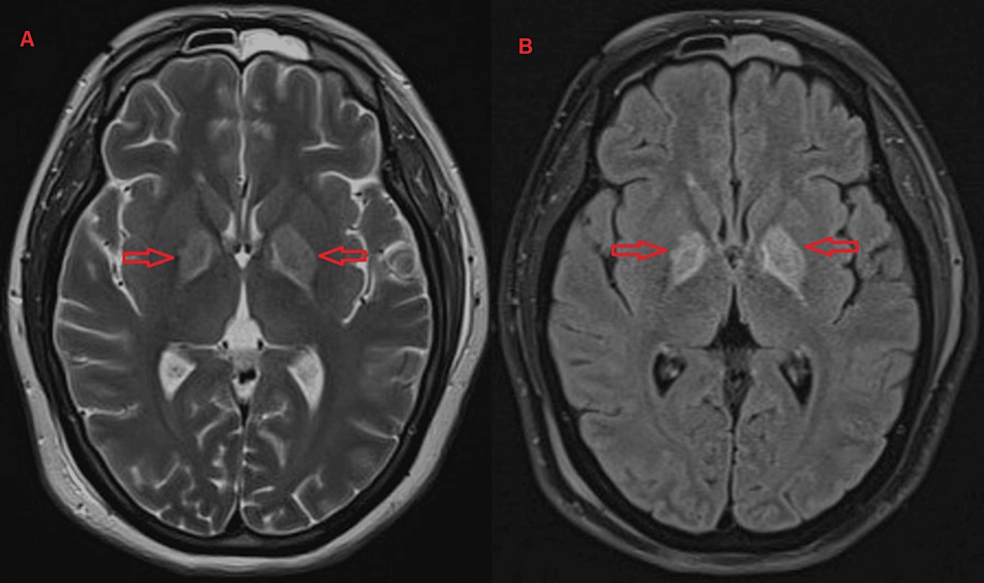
Magnetic resonance imaging (MRI) of the brain of Case 5 MRI brain of Case 5 showing bilateral symmetrical abnormal signal intensity involving the globus pallidi and, to a lesser extent, the centrum semiovale on both sides showing high signal intensity (red arrow) on T2 (2A) and fluid-attenuated inversion recovery (FLAIR) (2B)
